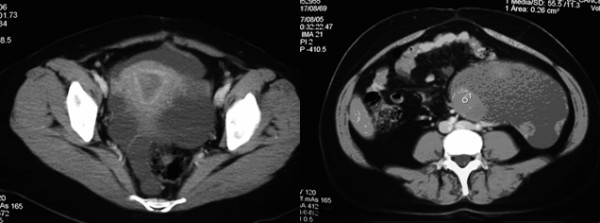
Computer tomography (CT) scan of pelvis. a). showing normal uterus and a pelvic mass located in the area of the left adnexa. b). showing cystic mass with solid areas.
